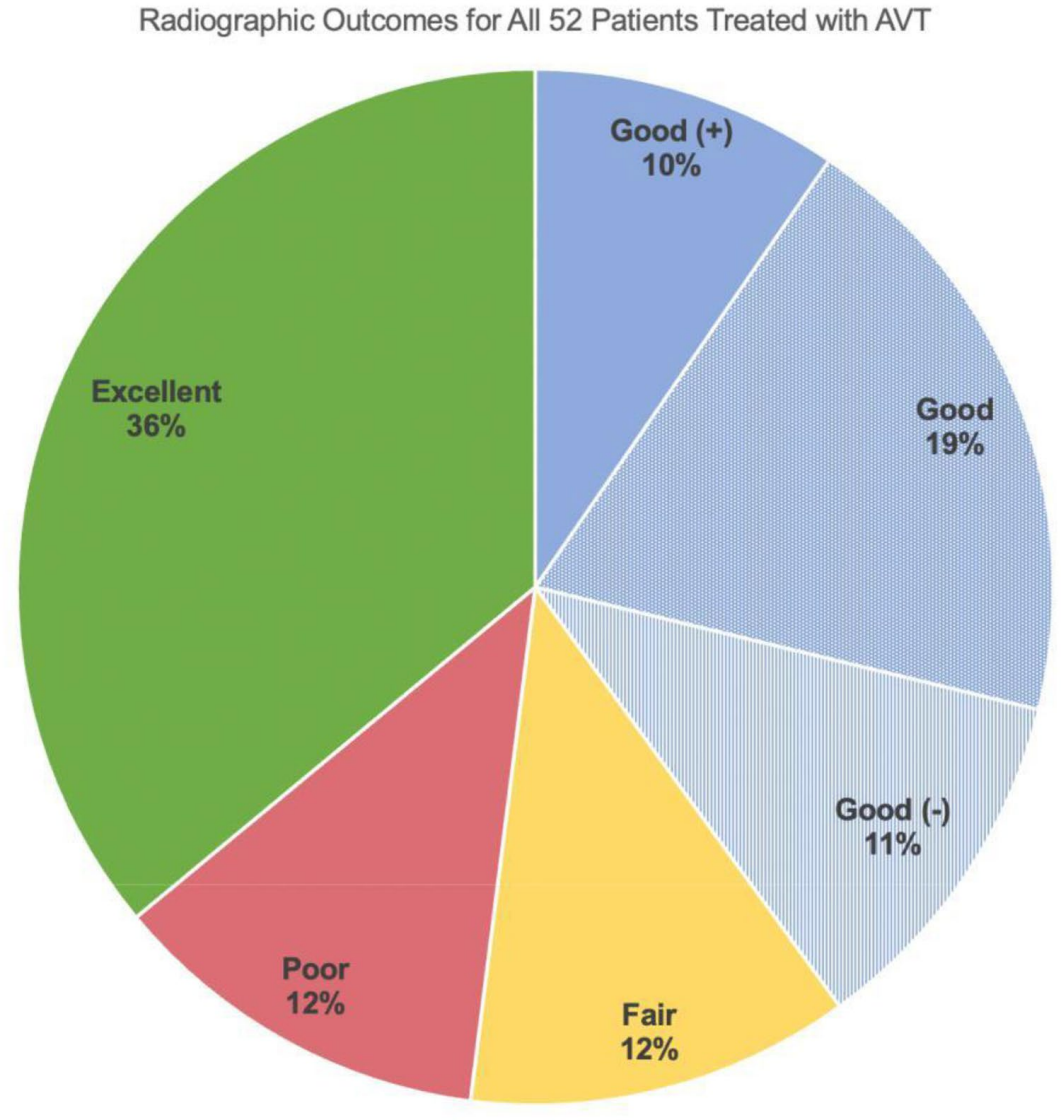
Radiographic outcomes were graded using final curve magnitude in all 52 patients with 74 curves treated with AVT: excellent (≤ 25°), good(+) (26–29°), good (30–35°), good (−) (36–39°), fair (40–49°), and poor (≥ 50°)
